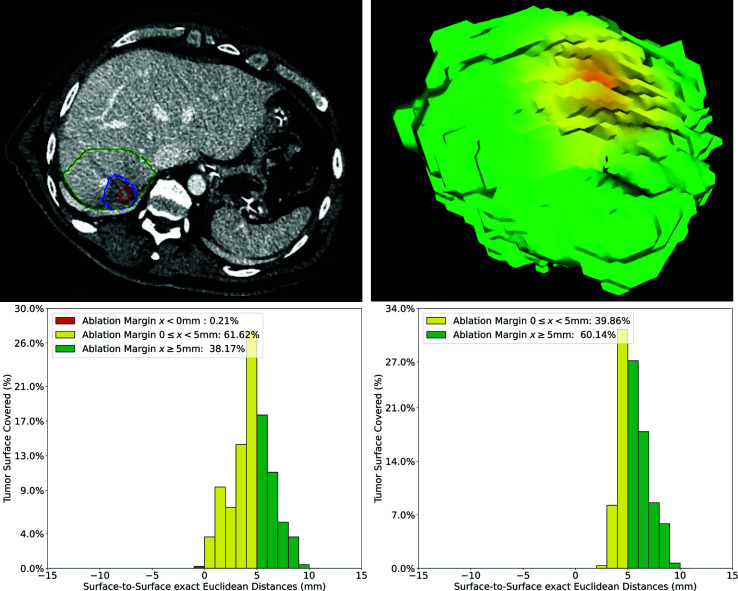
Volumetric assessment of quantitative ablation margins (QAM) of a subcapsular CRLM in liver segment VII. Upper left: Computed tomogram with segmented tumor (red), ablation (blue) volumes and the local liver capsule surrounding the tumor (green). Upper right: 3D representation of the ablation margin projected onto the ablation surface. Lower left: Histogram of ablation margin substrata without applying algorithm for subcapsular tumors. Lower right: Relative frequencies of ablation margin substrata with respect to percentage of tumor surface covered when applying algorithm for subcapsular tumors.
